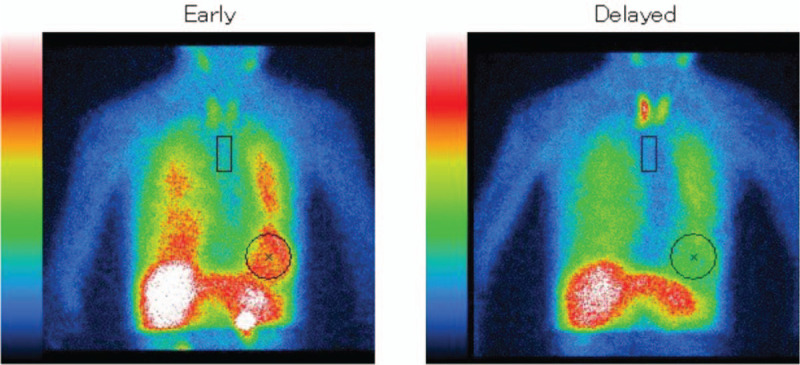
Cardiac meta-iodobenzylguanidine scintigraphy. Reduced accumulation of meta-iodobenzylguanidine indicated by the heart-to-mediastinum ratio is seen in the delayed phase.
